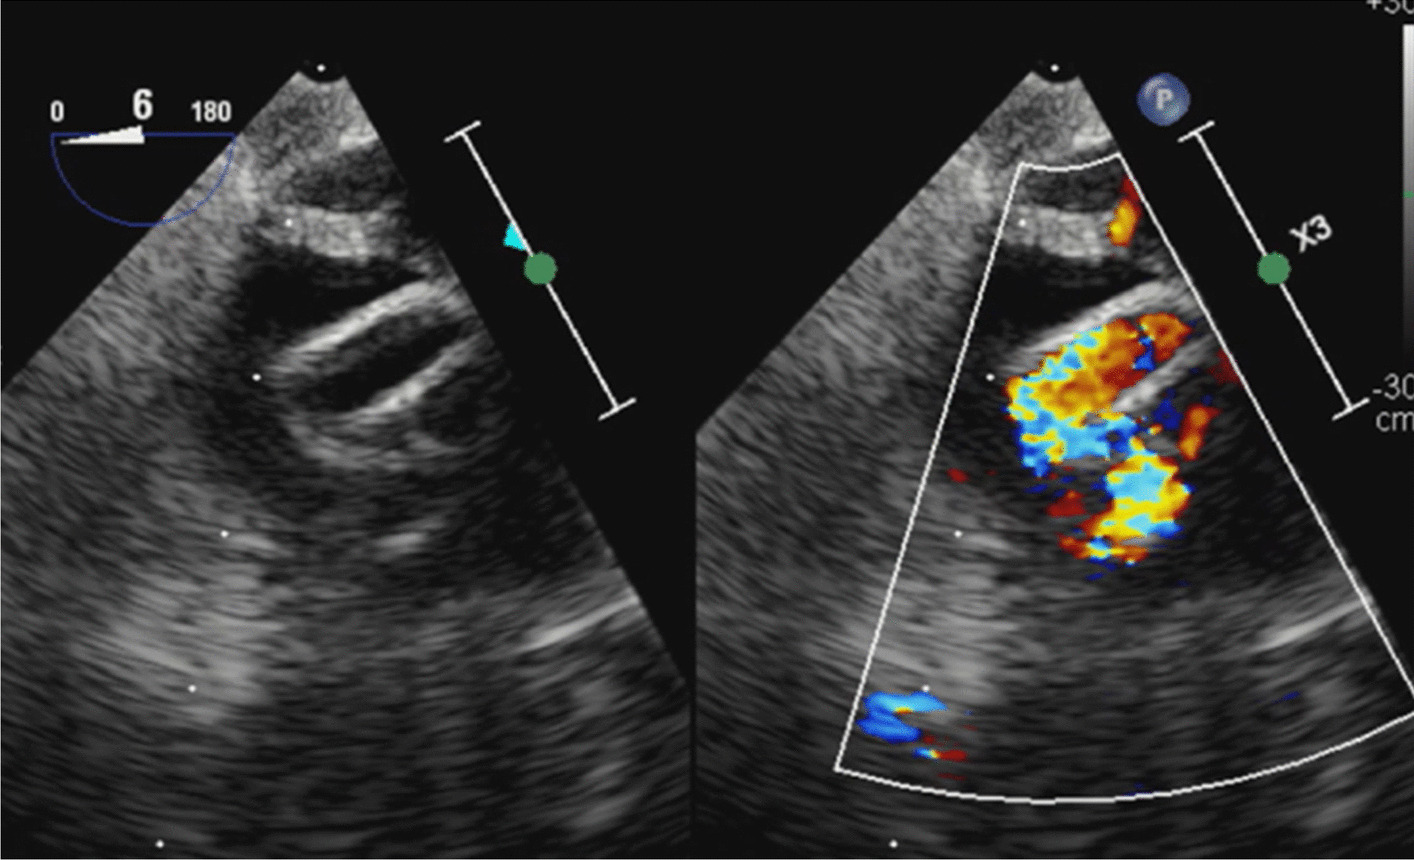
Transesophageal echocardiography (TEE) after an arterial cannula was added to the left axillary artery. After arterial cannula was added to the left axillary artery, the stenosis of the distal skirt of the Partial ET was improved
